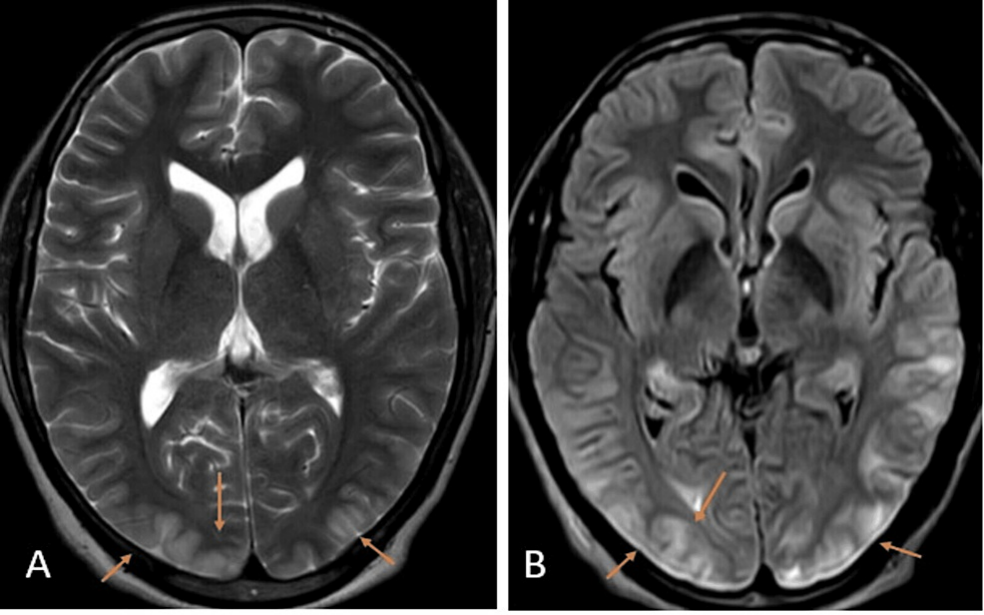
MRI of the brain axial sections T2-weighted imaging (A) and fluid-attenuated inversion recovery sequence (B) showing hyperintensities within the cortex and subcortical region of the bilateral occipital region with associated gyral swelling (orange arrows) suggesting posterior reversible encephalopathy syndrome.
